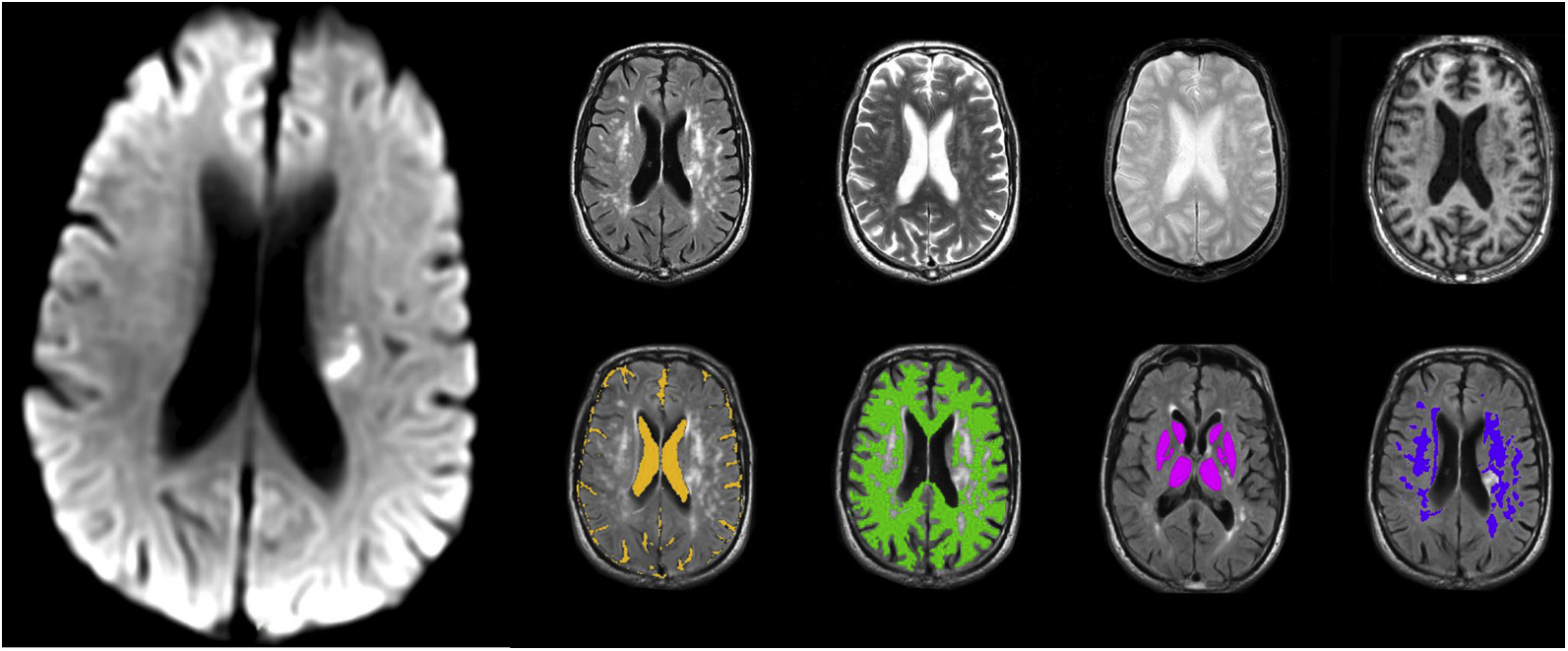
Magnetic resonance brain imaging sequences and processed images. Left, diffusion-weighted image shows recent small deep infarct in the left centrum semiovale (white area) as the index infarct. Top row, left to right, fluid-attenuated inversion recovery and T2-, T2*-, and T1-weighted axial brain images at the same level as in the large diffusion-weighted image on the left. Bottom row, left to right, colors indicated masking obtained by semiautomated image processing to identify cerebrospinal fluid (yellow), normal-appearing white matter (green), deep gray matter (pink), and white matter hyperintensities (purple); note the index infarct was masked by hand.
